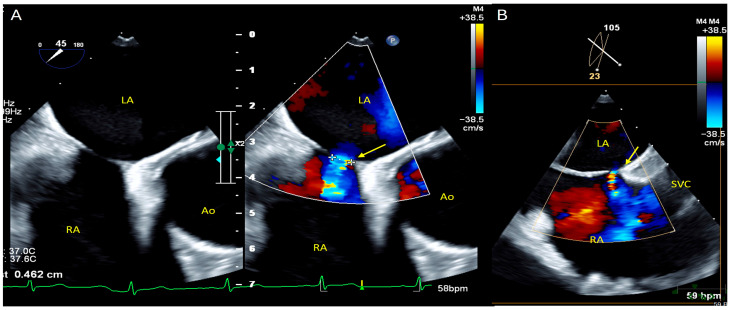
Transesophageal echocardiography confirming an ostium secundum atrial septal defect. Transesophageal echocardiography (TEE) was subsequently performed to confirm and characterize the anatomical nature of the intracardiac shunt. While transthoracic echocardiography serves as an effective initial screening tool, TEE is considered the gold-standard imaging modality for the definitive identification and characterization of interatrial communications, including patent foramen ovale and atrial septal defects [6,8,9]. The examination was performed using an X8-2t transesophageal probe (xMatrix TEE, Philips Medical Systems, Andover, MA, USA). Transesophageal echocardiography in the short-axis view (A) demonstrated an ostium secundum atrial septal defect measuring approximately 4.6 mm, shown on grayscale imaging ((A), left panel) and with bidirectional flow on color Doppler ((A), right panel). The defect was further visualized measuring approximately 4.6 mm on transesophageal echocardiography in the bicaval view (B), clearly delineating the atrial septal defect (yellow arrow). Isolated atrial septal defects, as observed in this case, are classified as simple congenital heart defects in which a deficiency of the atrial septum allows abnormal interatrial communication. They account for approximately 6–10% of all congenital heart defects and represent the most common acyanotic congenital cardiac lesion [10]. The estimated prevalence of atrial septal defects in adults is approximately 0.88 per 1000 individuals [11]. Atrial septal defects are classified into four anatomical subtypes: ostium secundum, ostium primum, sinus venosus, and coronary sinus defects. Ostium secundum defects constitute approximately 80% of all atrial septal defects and represent the only true deficiency of the fossa ovalis region, without involvement of adjacent cardiac structures or atrioventricular valves [12]. Ao = aorta; LA = left atrium; RA = right atrium; SVC = superior vena cava.
